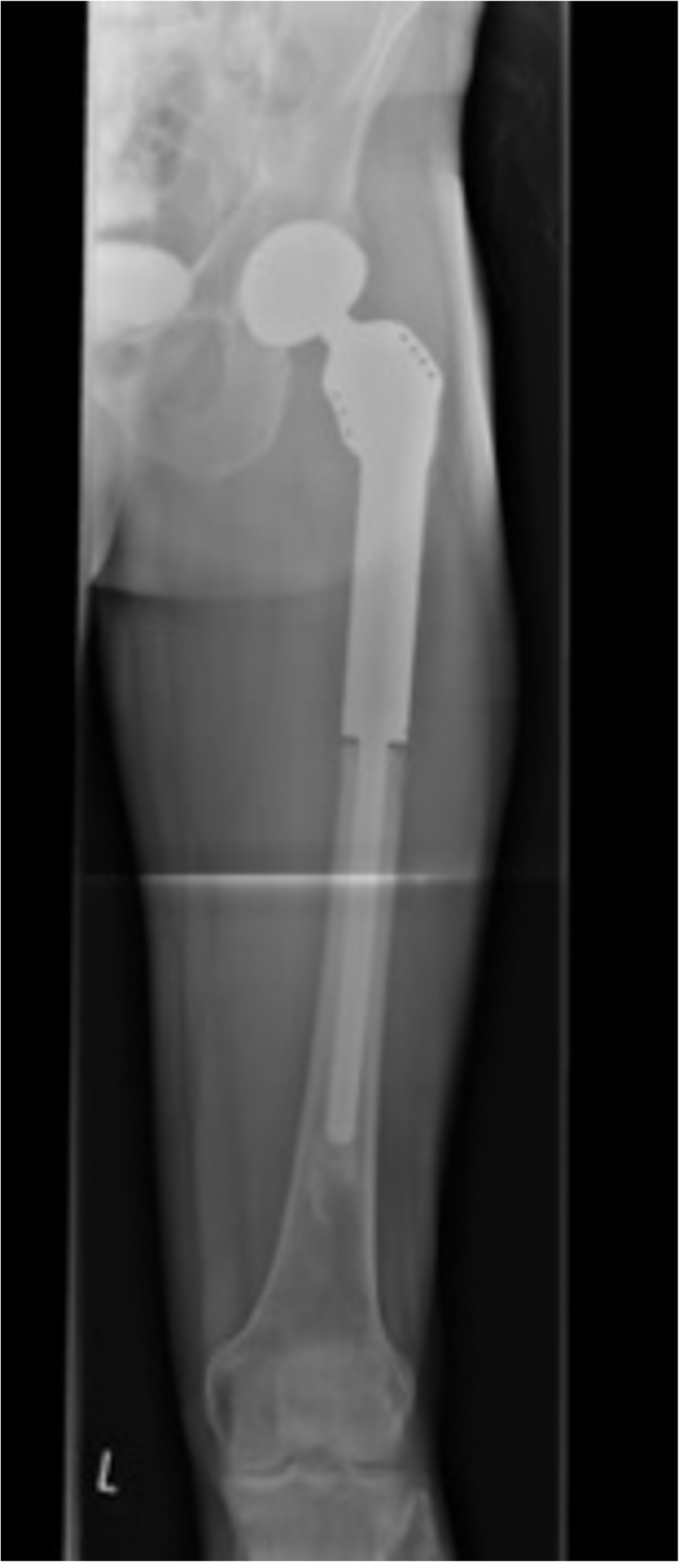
X-ray showing the Modular Munich-Luebeck (MML) femoral megaprosthesis in situ
